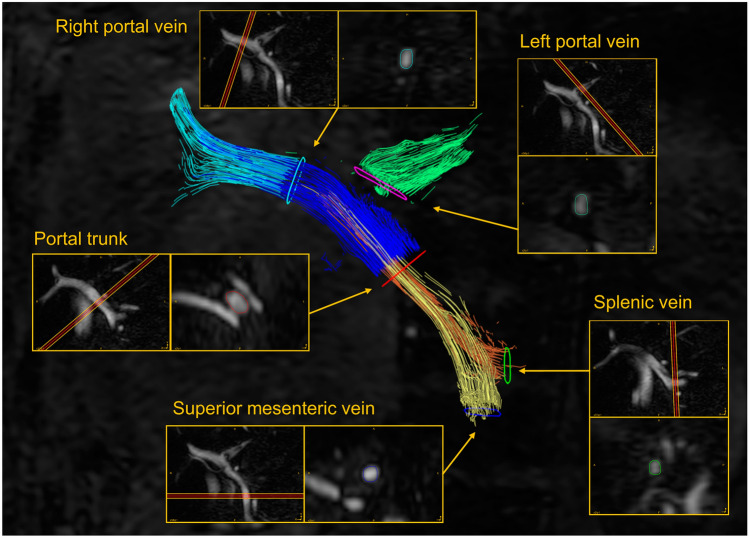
Velocity map of four-dimensional (4D) flow MRI of a 46-year-old female patient with normal liver (Fib-4 index = 0.39, APRI = 0.16). The 4D flow MRI velocity map shows the flow in the portal trunk (PT, dark blue), right portal vein (RPV, light blue), left portal vein (LPV, green), superior mesenteric vein (SMV, yellow), and splenic vein (SV, orange). For 4D blood flow analysis, the radiologists manually defined the region of interest on the PT, RPV, LPV, SMV, and SV cutting planes. Abbreviations: *APRI* aspartate aminotransferase-to-platelet ratio index, *Fib-4 index* fibrosis-4 index, *MRI* magnetic resonance imaging
